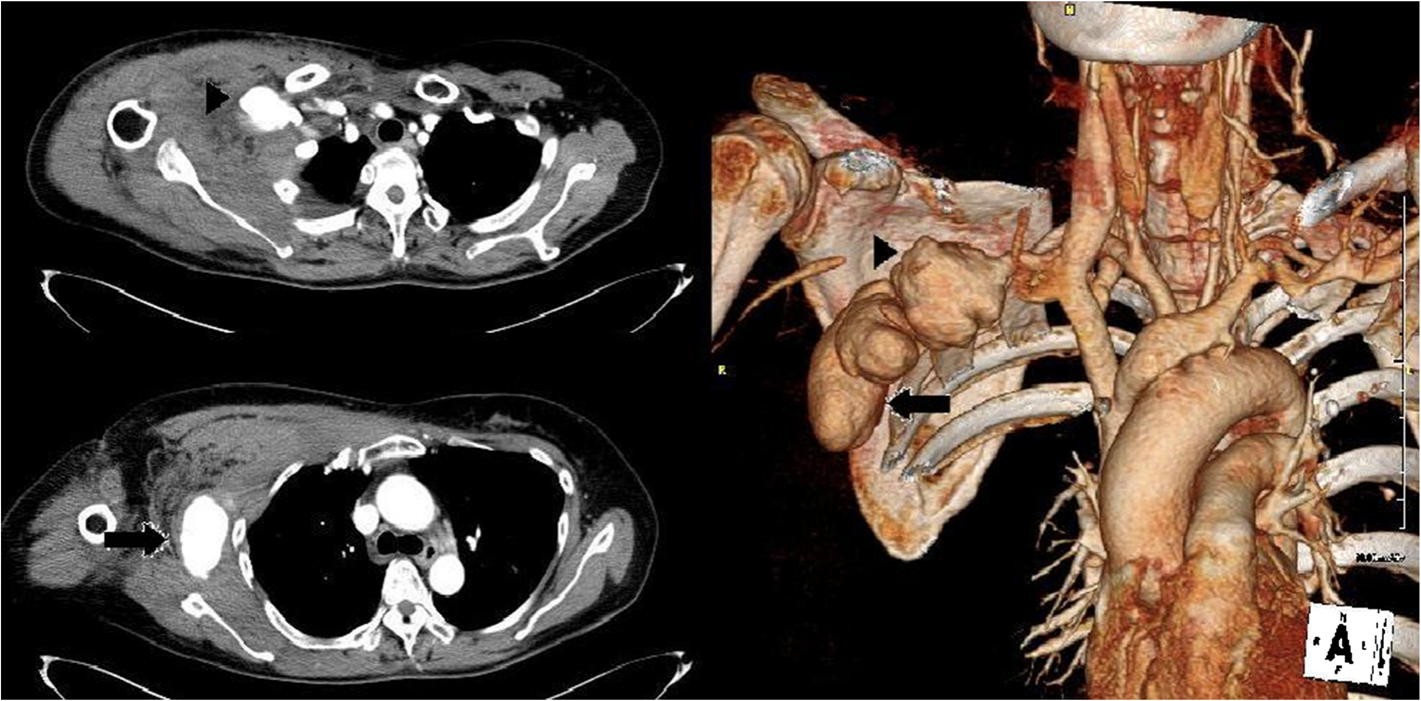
Contrast-enhanced CT scan shows a pseudoaneurysm (arrow head) of the right subclavian artery with a downward protruding pseudoaneurysm (arrow).
